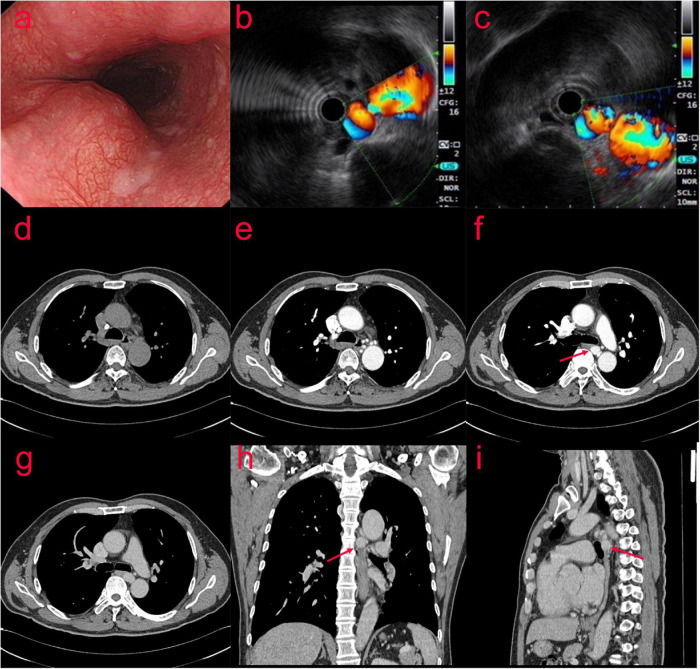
(Case one) The patient with Bronchial artery aneurysm (BAA) was examined by gastroscopy (a) at the local hospital, which showed an “esophageal mass.” After visiting our hospital, ultrasonic gastroscopy showed an esophageal bulge, vascular compression, and suspected BAA (b,c). A contrast-enhanced CT scan showed multiple tortuous and thickened vascular shadows (maximum width about 1.3 cm) in the middle and posterior mediastinum and around the esophagus (d–i).
